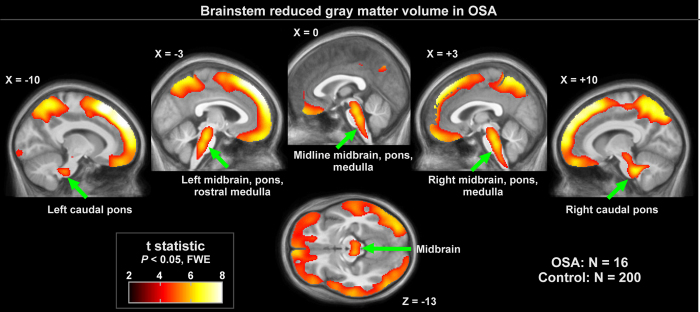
Brainstem regions of significantly lower regional grey matter volume in OSA (n = 16) over control (n = 200) subjects (*P* < 0.01), colored according to significance level. Background is the average of the 216 subjects’ normalized T1-weighted brain scans. Slice locations are in MNI coordinates.
